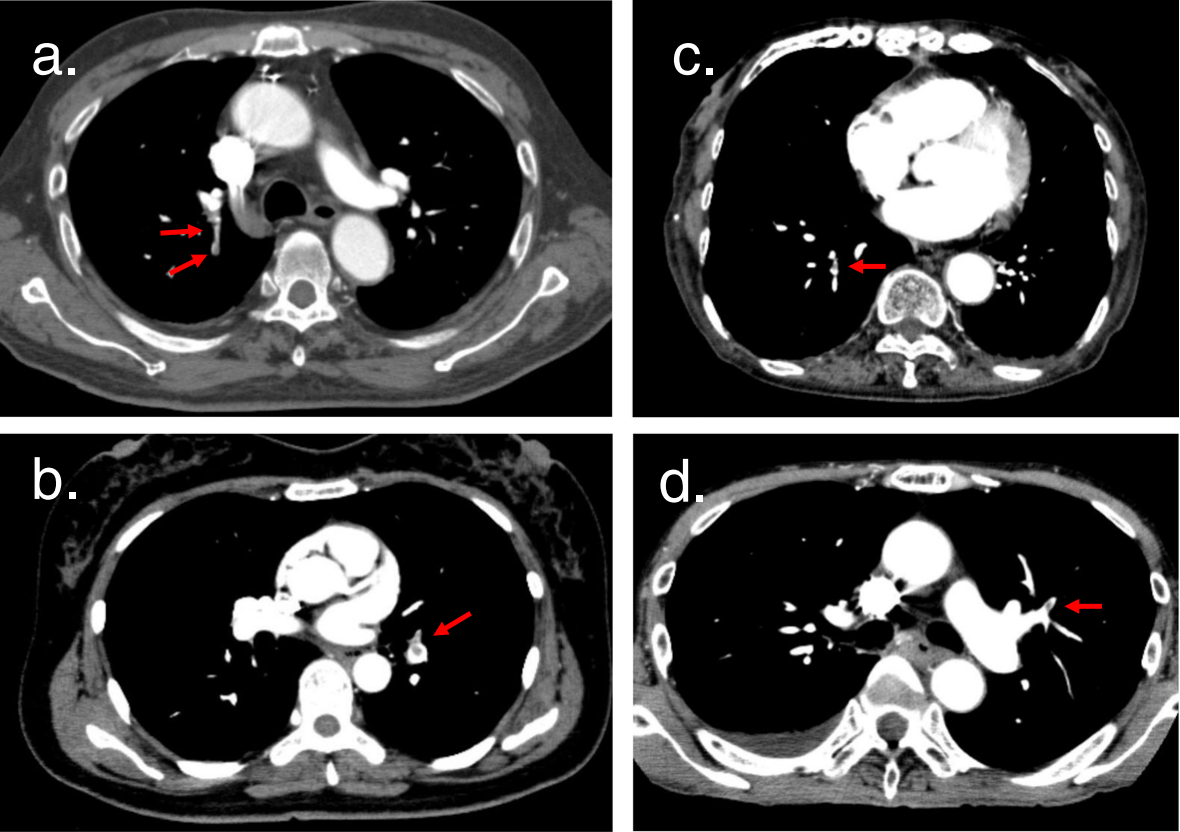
Enhanced CT images of pulmonary embolism in four patients with acute appendicitis. Red arrows indicate thrombi. a On POD3, plasma D-dimer level was elevated to 23.3 μg/ml. Enhanced CT revealed thrombus in right pulmonary artery. DVT was not found. b On POD6, the patient complained of right lower leg pain. Enhanced CT was performed without D-dimer test. Embolism in the left pulmonary artery and thrombi in the right popliteal vein and the right soleal vein were detected. c On POD6, plasma D-dimer level was elevated to 48.0 μg/ml. Some embolus was detected on both sides of the peripheral pulmonary arteries. DVT was detected in the left soleal vein. d On POD5, plasma D-dimer level was elevated to 33.2 μg/ml. Pulmonary embolism was detected in the upper right pulmonary artery. DVT was detected in the right popliteal vein. CT, computed tomography; POD, postoperative day; DVT, deep venous thrombosis
